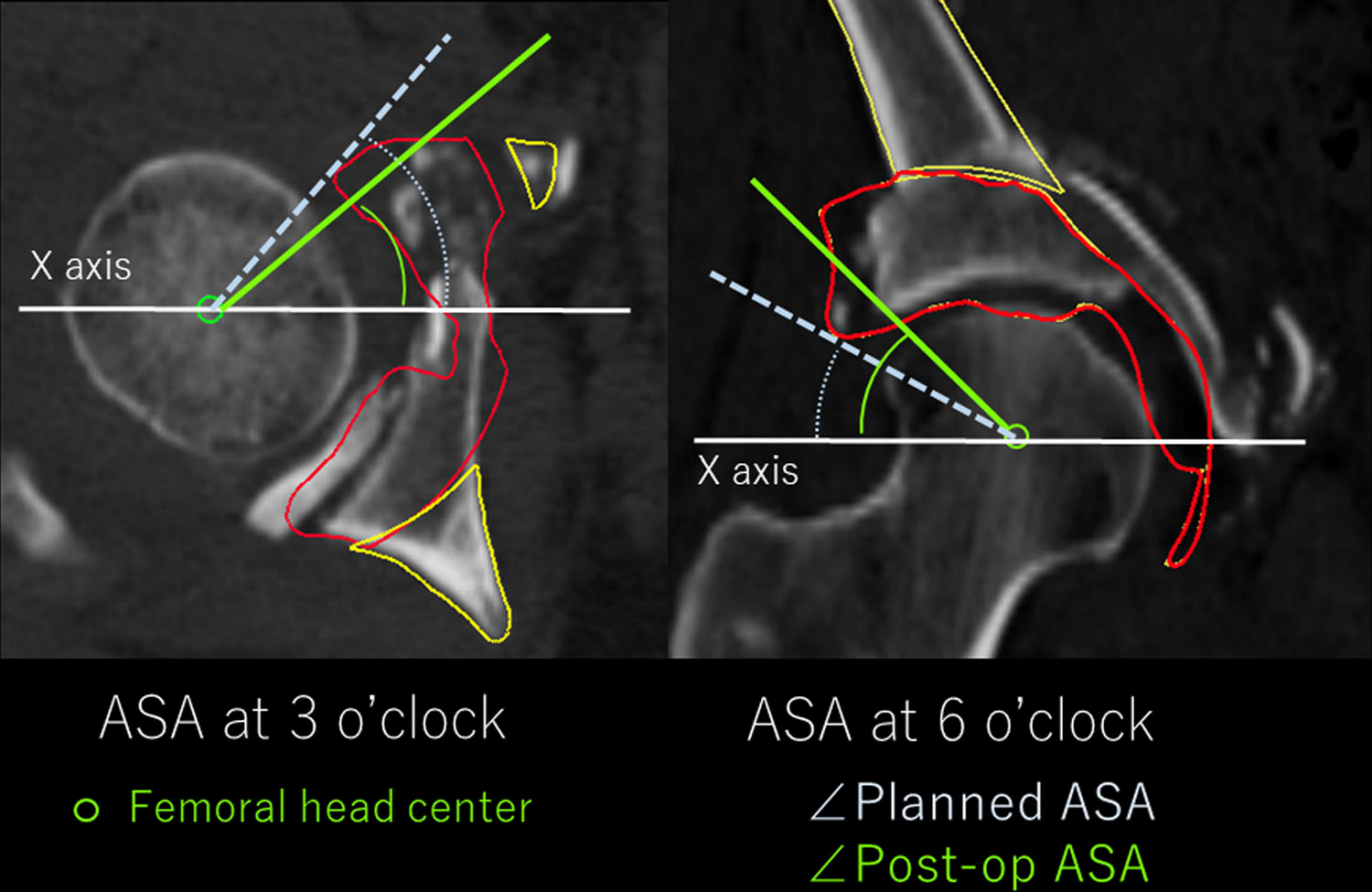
Definition of acetabular sector angle (ASA): index of acetabular coverage. The ASA was defined as the angle between the line drawn from the acetabular rim to the femoral head center and the *X*-axis on every radial reconstruction image. Figure shows the ASA at the 3:00 position using postoperative CT superimposed with the planning model. The red line indicates the planning model. The angle made by the *X*-axis and the line connecting the femoral head center and acetabular rim in planning (dotted line) is the planned ASA. The angle made by the X-axis and the line connecting the femoral head center and actual post-operative acetabular rim (green line) is the postoperative ASA. a Shows the ASA at 3 o’clock and b shows the ASA at 12 o’clock. The difference between the planned and postoperative ASAs is denoted as ΔASA, i.e., the index of accuracy in acetabular fragment reorientation. If the ΔASA is positive, it means that the postoperative acetabular coverage exceeds the preoperative plan. Conversely, if the ΔASA is negative, it implies under-correction compared to the preoperative plan
